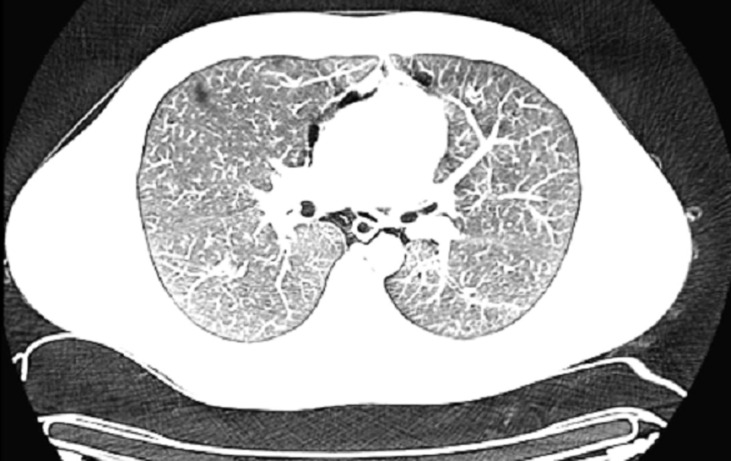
chest CT scan: axial view showing pneumomediastinum and a thin rim of pneumothorax
